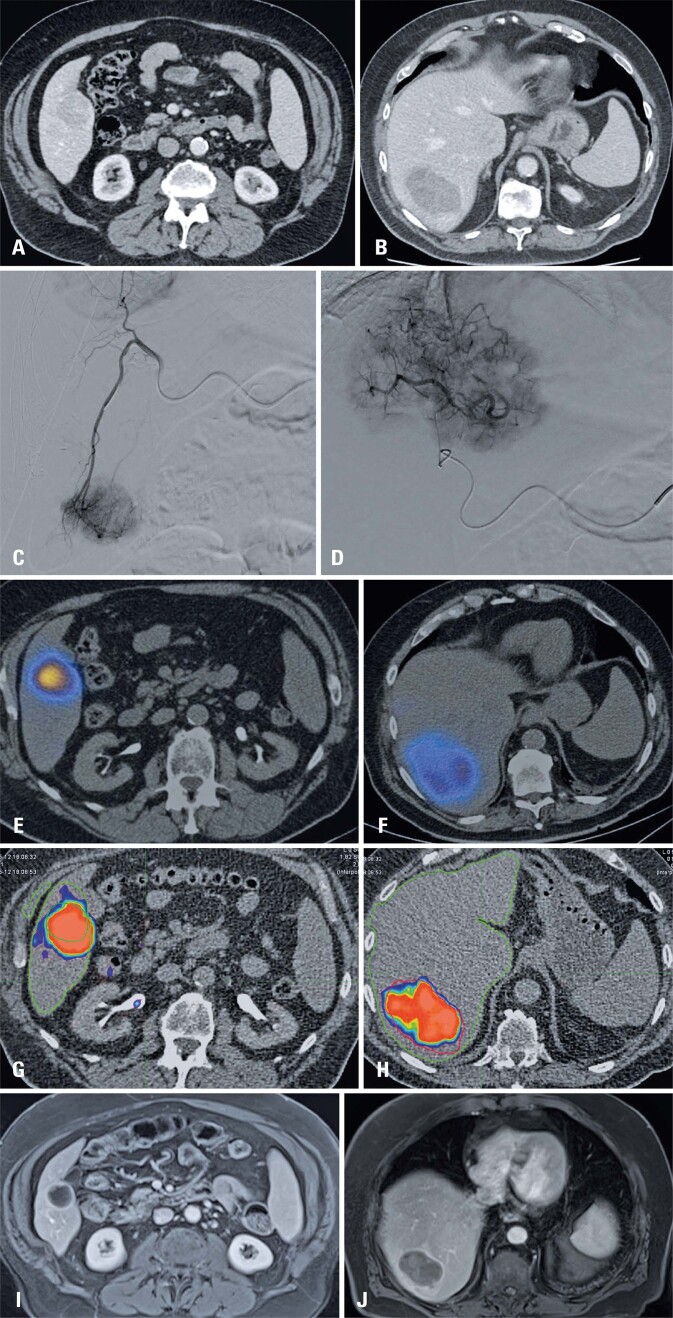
Case of a patient who showed an objective response: A to B) Pretreatment abdominal tomography identifying: Lesion 1: Segment V, near the gallbladder bed, measuring 4.1×3.6cm, hypervascularized with washout (LR-5) and Lesion 2: Segment VII, in contact with the diaphragmatic surface of the liver, measuring 9.5×5.9cm, hypervascularized with washout (LR-5). C) Selective angiography showing a hypervascular lesion in segment V. D) Selective angiography showing a hypervascular lesion in segment VII. E) SPECT-CT following injection of macro-aggregated albumin confirming adequate uptake in the target lesion of segment V, with a hepato-pulmonary shunt of 9.5% and absence of extra-hepatic uptake. The administered activity was 0.8 GBq. F) SPECT-CT after injection of macro-aggregated albumin confirming adequate uptake in the target lesion of segment VII. The administered activity was 2.1 GBq. G) Reconstruction of the Y90 PET in MIM software demonstrating the uptake of the target lesion in segment V, with a strong red color scale for 200Gy and dose reduction in cooler colors. H) Reconstruction of the Y90 PET in MIM software demonstrating the uptake of the target lesion in segment VII, with a strong red color scale for 200Gy and dose reduction in cooler colors. I) Magnetic resonance imaging 90 d post-procedure showing treated hepatic nodules of segment V measuring 2.9cm (average, 3.7cm), predominantly necrotic with thin internal septa and hypervascular enhancement ≤0.3cm thick. J) Magnetic resonance imaging 90 d post-procedure showing the periphery of segment VII measuring 5.5cm (was 7.5cm), with internal hemorrhagic foci, central necrotic area, and solid hypervascular peripheral portion measuring up to 4.8cm (average, 6cm)
